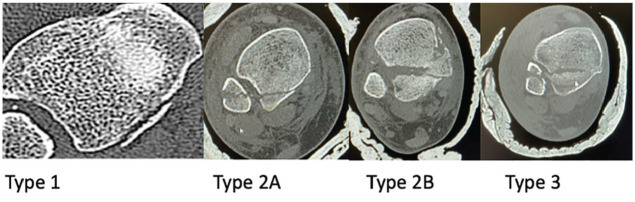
Axial CT scan showing different types of posterior malleolar fractures based on the Mason and Molloy classification.
